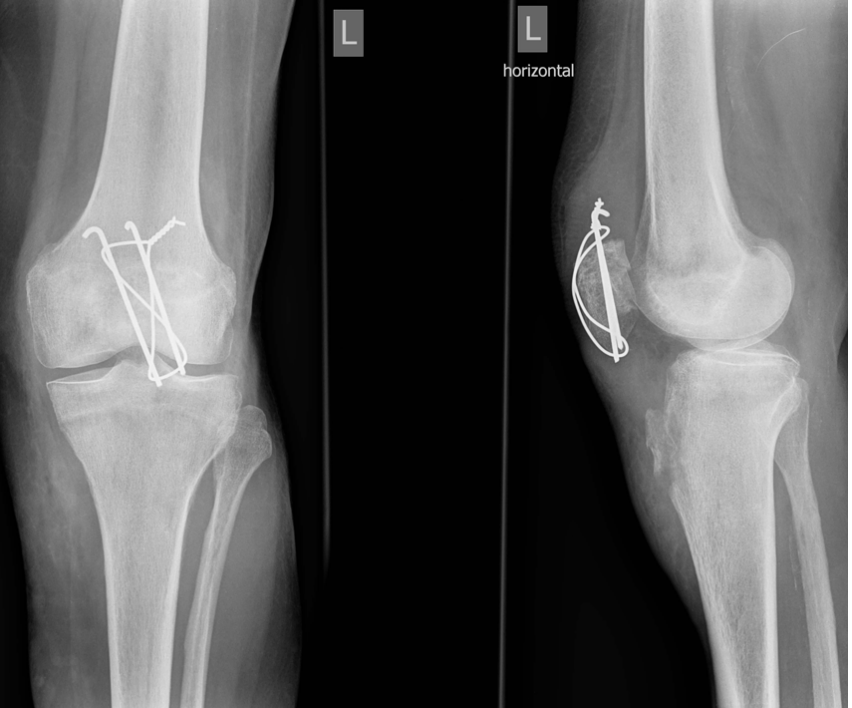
Anteroposterior and lateral radiographs of left knee showing the avulsion fracture of the tibial tuberosity, a subtle proximalisation of the K-wires and intraarticular step due to a bony defect.
